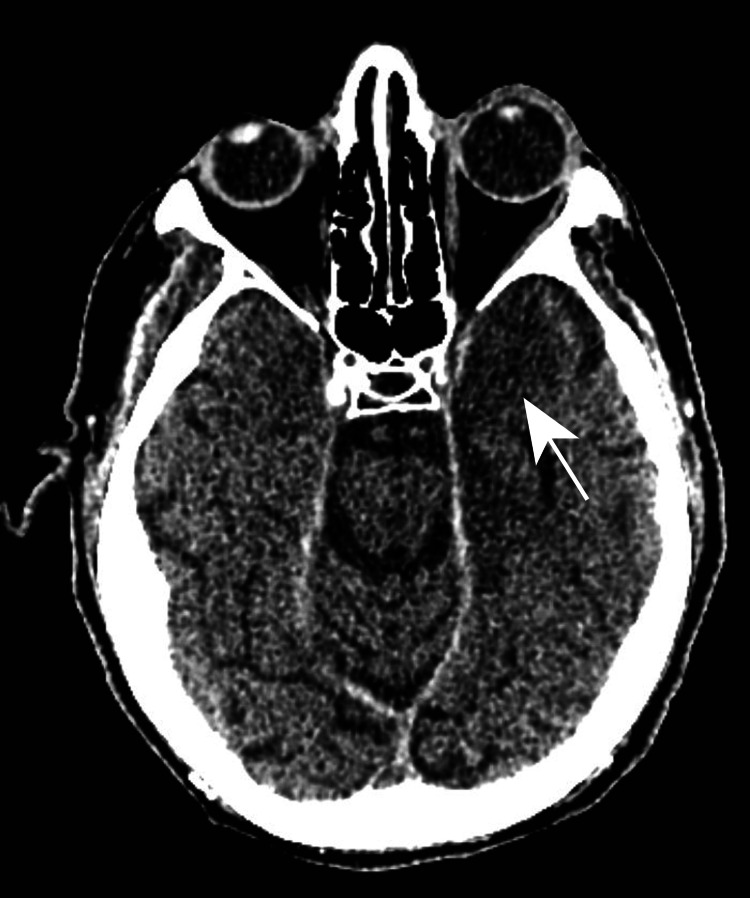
CT scan of the brain with hypodensity in the left anterior temporal pole
